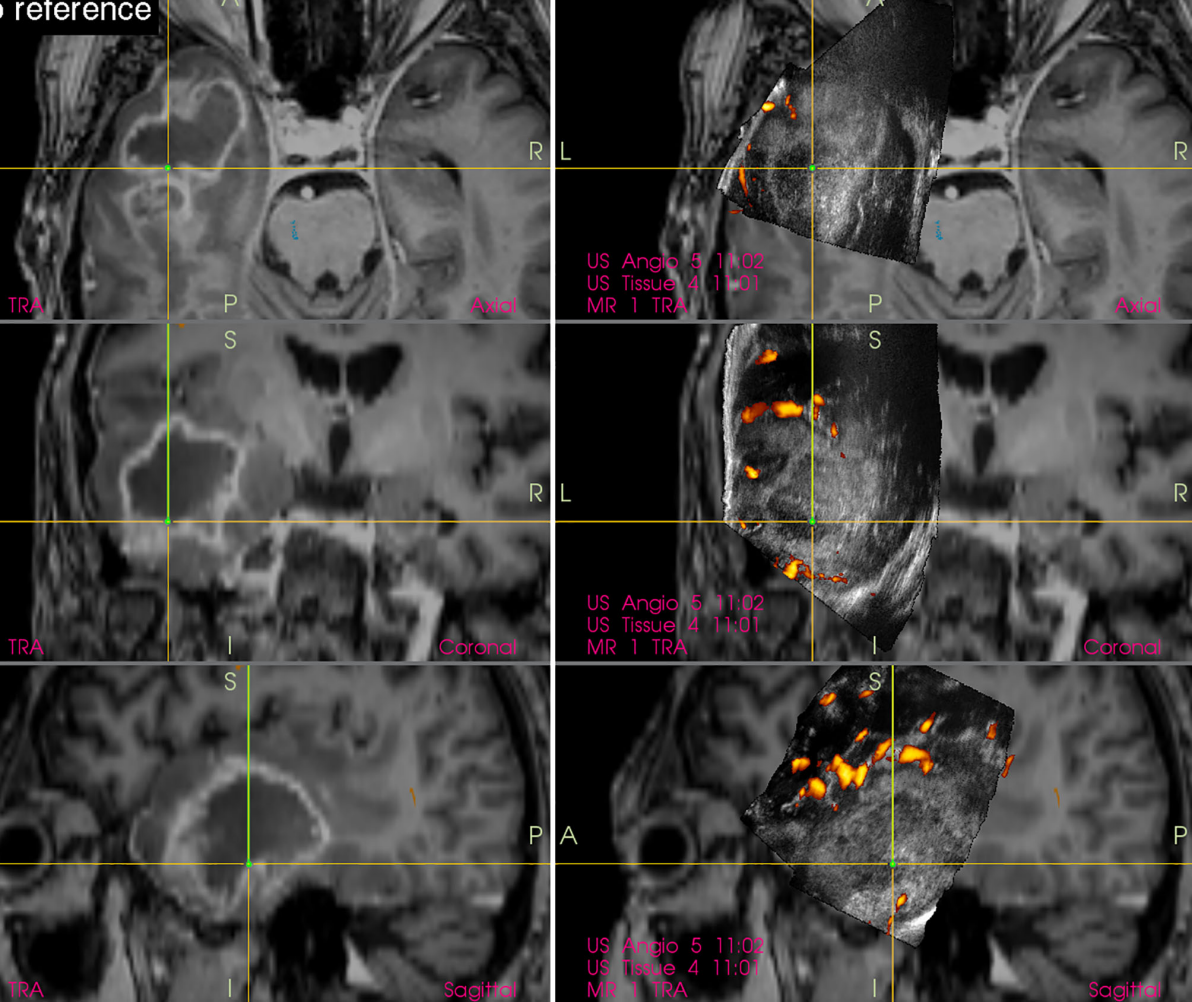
Right-sided temporal glioblastoma (Left column) preoperative navigation 3D contrast-enhanced T1-weighted MRI sequence displaying high-grade tumor showing typical ring enhancement (Right column) pre-resectional 3D IOUS image fused with navigation MRI. Note that the hyperechoic high-grade tumor tissue is not selectively identifiable on the IOUS image, as the surrounding edematous and infiltrated brain (non-enhancing on MRI) is hyperechoic as well. 3D, three-dimensional; MRI, magnetic resonance imaging; IOUS, intraoperative ultrasound.
